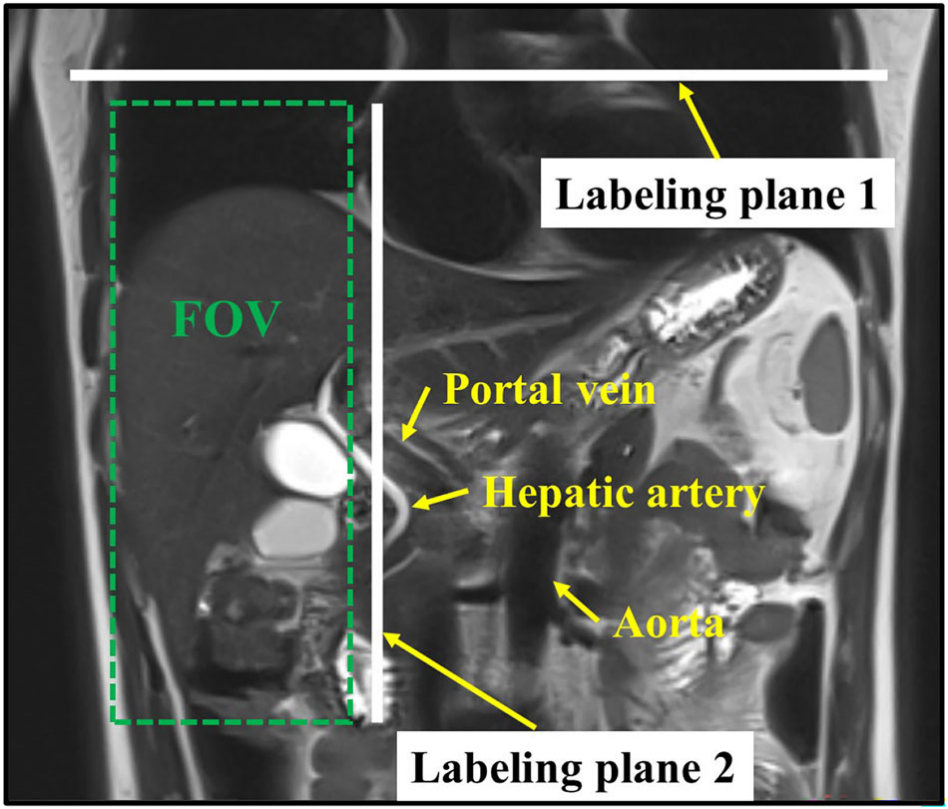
Field of view (FOV) and two labeling planes of ASL-MRI
